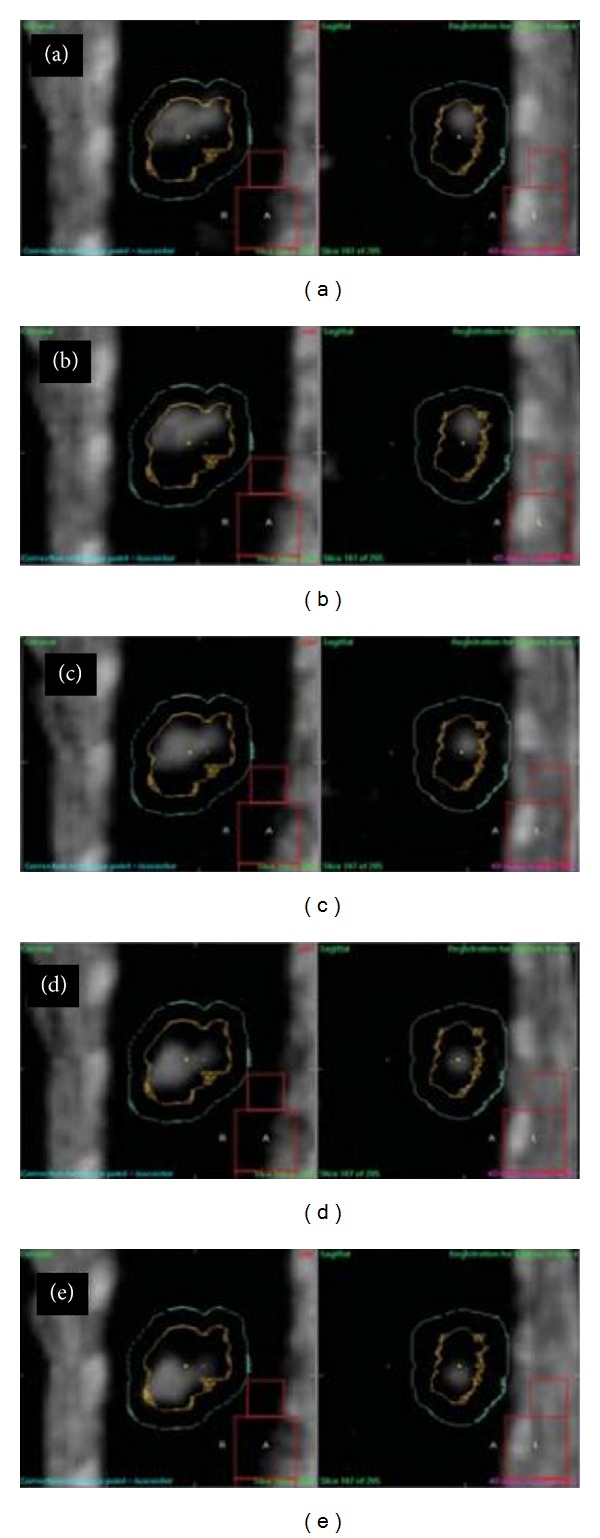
4D CBCT images on the first day overlaid with PTV (in sky blue) and ITV (in yellow) contours after lung tumor registration for five consecutive respiratory phases covering half a breathing cycle, where the tumor moves from cranial to caudal direction during the half cycle [9].
